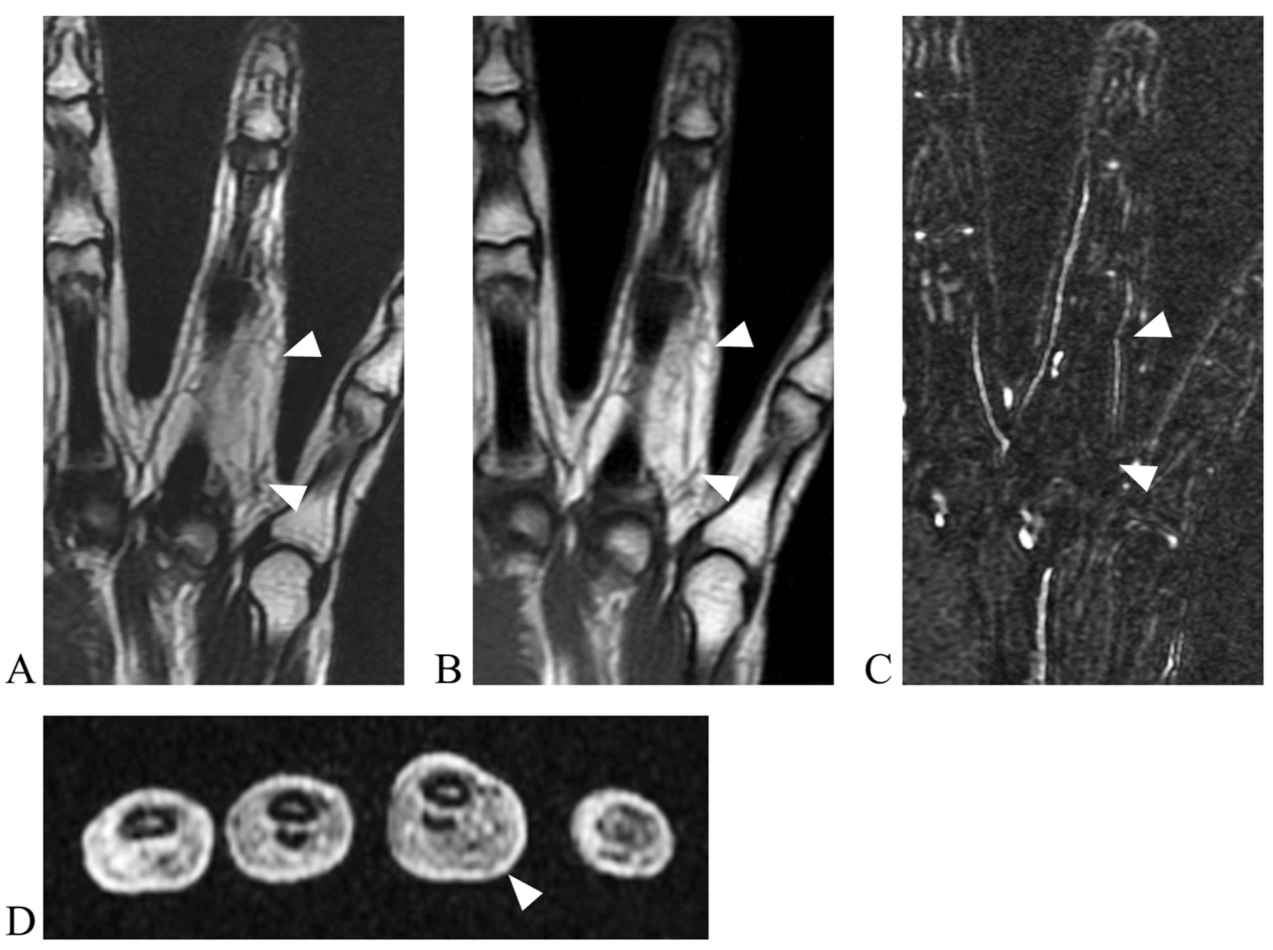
Magnetic resonance imaging demonstrating a fatty lesion on the ulnar side of the left ring finger (white arrow). (A) Sagittal section, T1-weighted images; (B) sagittal section, T2-weighted images; (C) sagittal section, fat-suppressed image; (D) axial section, T1-weighted images.
